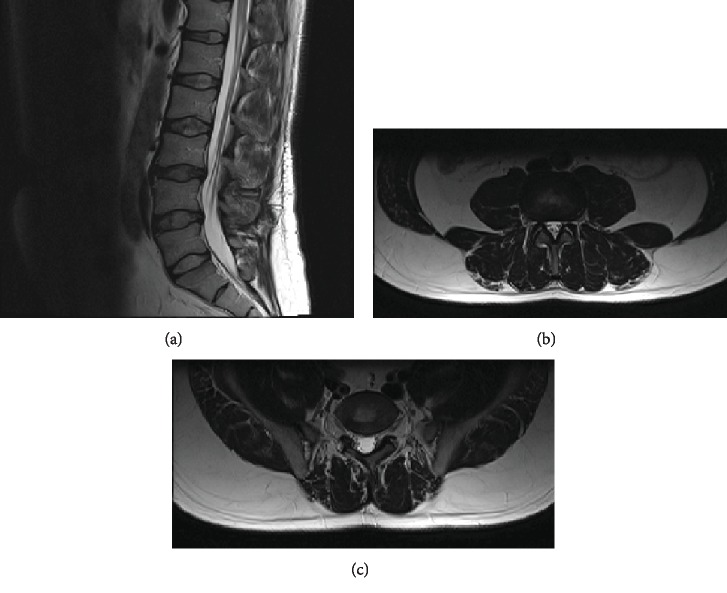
(a) Sagittal T2 lumbar spine MRI showing multiple disc bulges at L3/L4 and L4/L5. (b) Axial T2 at the L4/L5 level showing a mild disc bulge narrowing the right nerve root foramen. (c) Axial T2 at the L5/S1 level showing mild right paracentral disc bulge.
